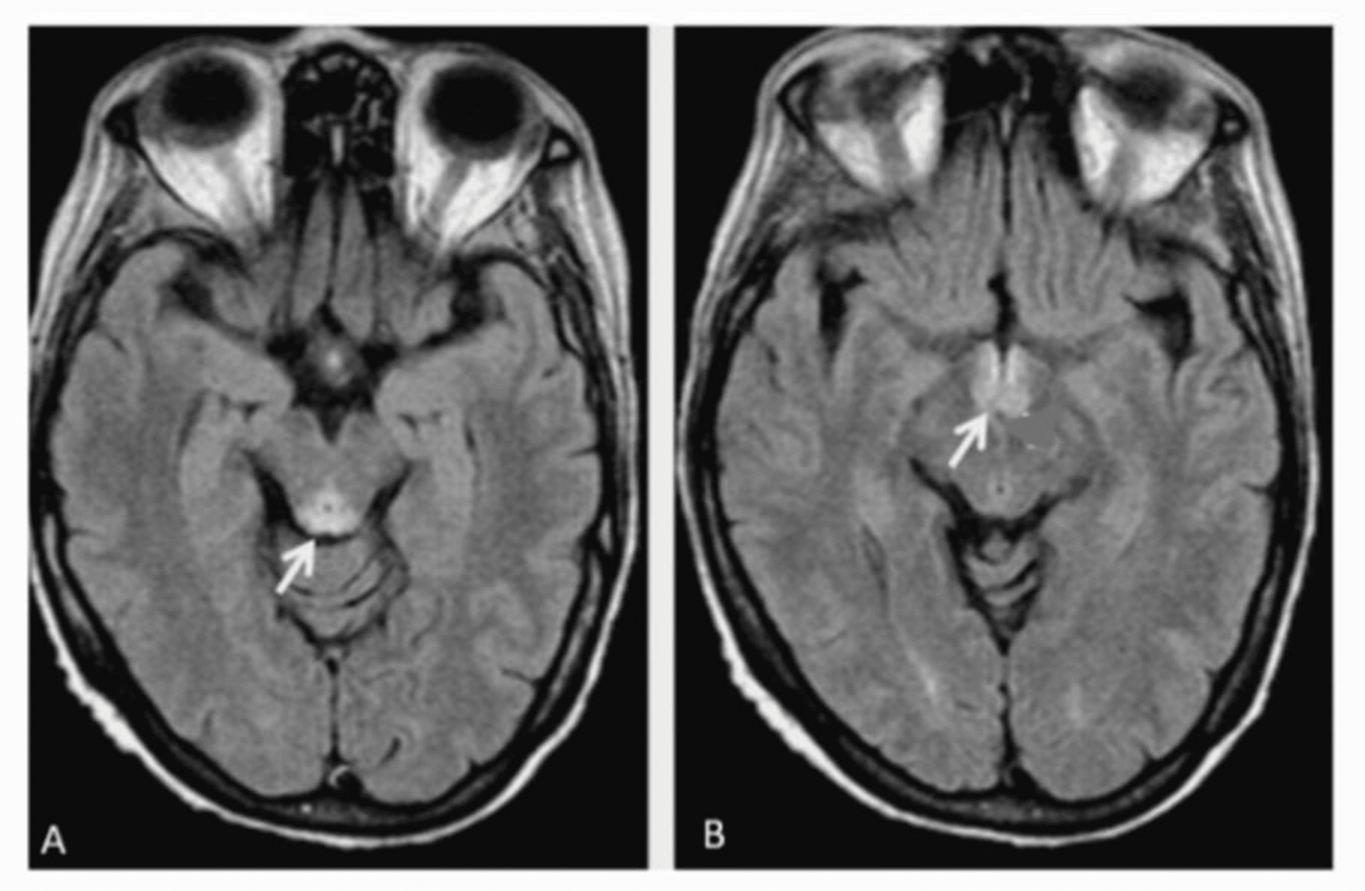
Brain MRI for Case 4 FLAIR images showing T2 hyperintensity in the periaqueductal gray (A) and mamillary bodies (B) as shown with arrows. MRI: magnetic resonance imaging, FLAIR: fluid-attenuated inversion recovery
